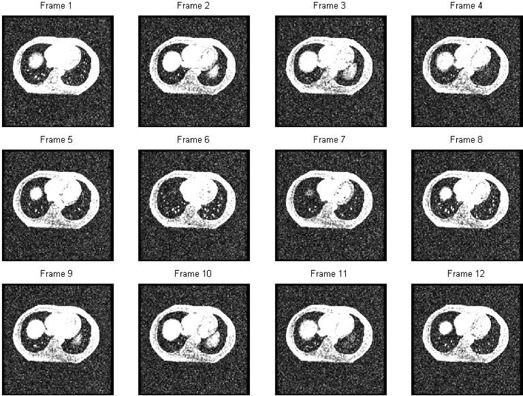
All 12 frames of one slice from the patient MR study acquired with the 2D FSPGR sequence. These frames are displayed at the signal intensity level of noise; motion ghosting artifacts that usually affect clinical MR images are not visible in these images, which were acquired under free‐breathing, indicating the effectiveness of the proposed MR protocol.
